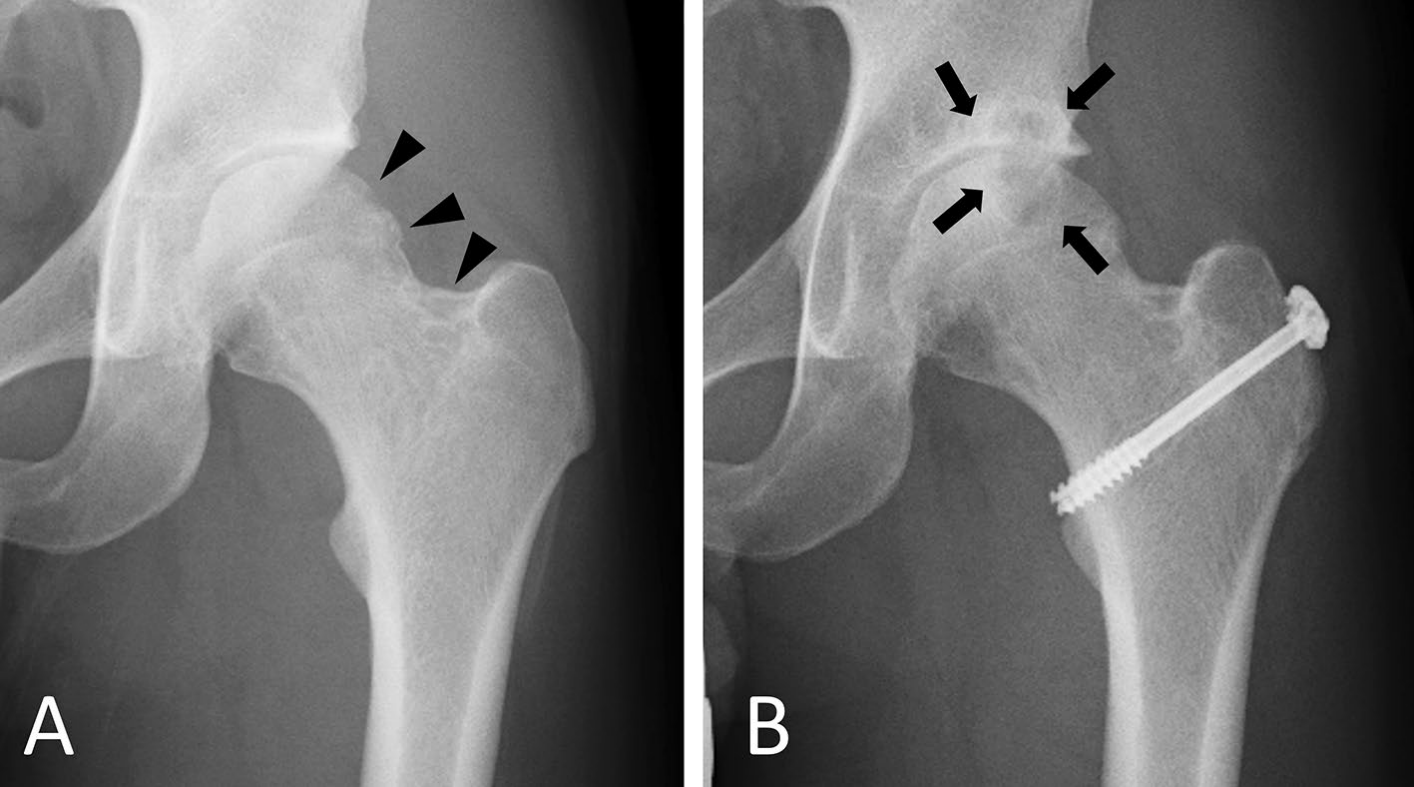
Osteochondral lesion in hip joint. (A) A 27-year-old men with hip TSGCT. An anterior–posterior radiograph showed osteolytic lesion in left femoral head and neck region (arrowheads), however there is no obvious osteoarthritic change at initial examination. (B) Three years after tumor resection. After surgery for TSGCT, osteoarthritic change occurred (arrows).
